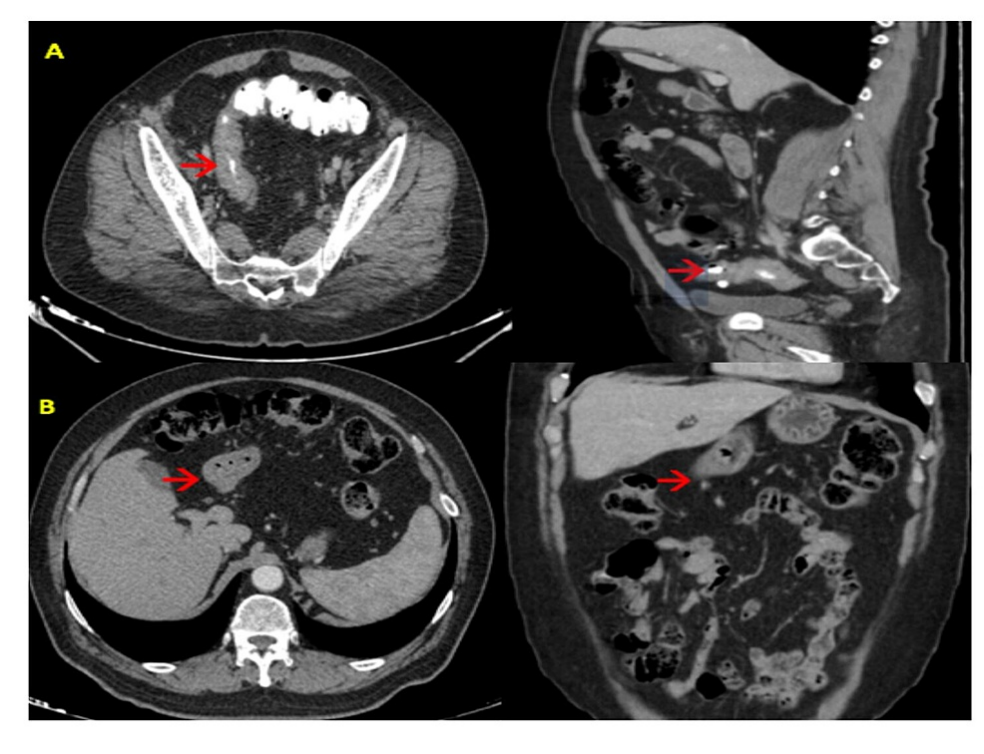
Preoperative CT findings. (A) Coronal and axial CT showed increased wall thickening of the sigmoid colon (red arrow). (B) Coronal and axial CT showed increased wall thickening of the gastric antrum (red arrow).
